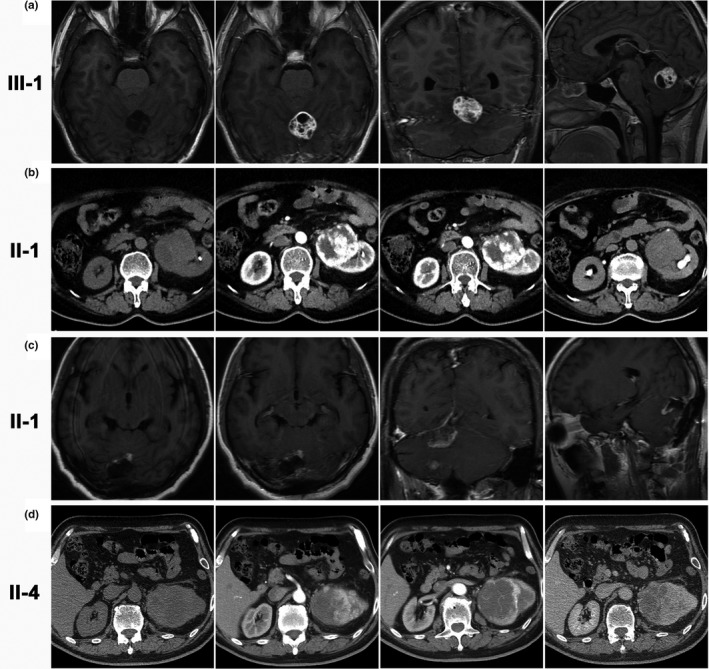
Radiological findings of the four tumors from the three patients. (a) and (c) Images of CH tissues from Patients III‐1 and II‐1 captured using brain MRI. (b) and (d) Abdominal CT images of the RCCC tissues from Patients II‐1 and II‐4. The CH was a mixed mass with both solid and cystic segments. The solid part displayed a isointense/hyperintense signal on T1‐weighted images; the cystic part displayed a hypointense signal. Contrast‐enhanced imaging of the axial, coronal and sagittal planes revealed that the signal of the solid part was markedly enhanced while the cystic part showed a similar hypointense signal to the plain scan. The RCCC originated from the kidney, showing mixed density on plain CT because of hemorrhaging and necrosis inside the fast‐growing tumor. The enhanced CT images revealed a homogenous enhancement in the solid part and no enhancement inside the tumor
